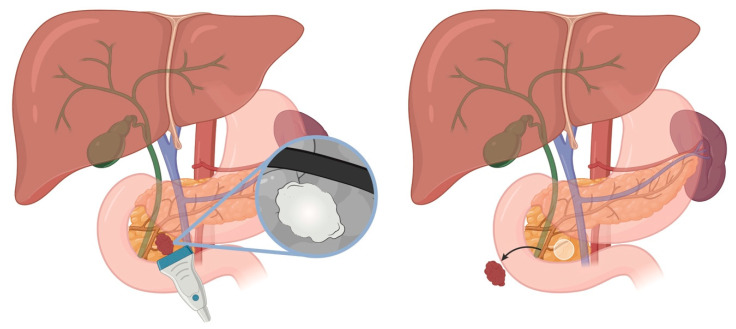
Intraoperative ultrasound identification of Insulinoma (sonolucent tumor within pancreas).
